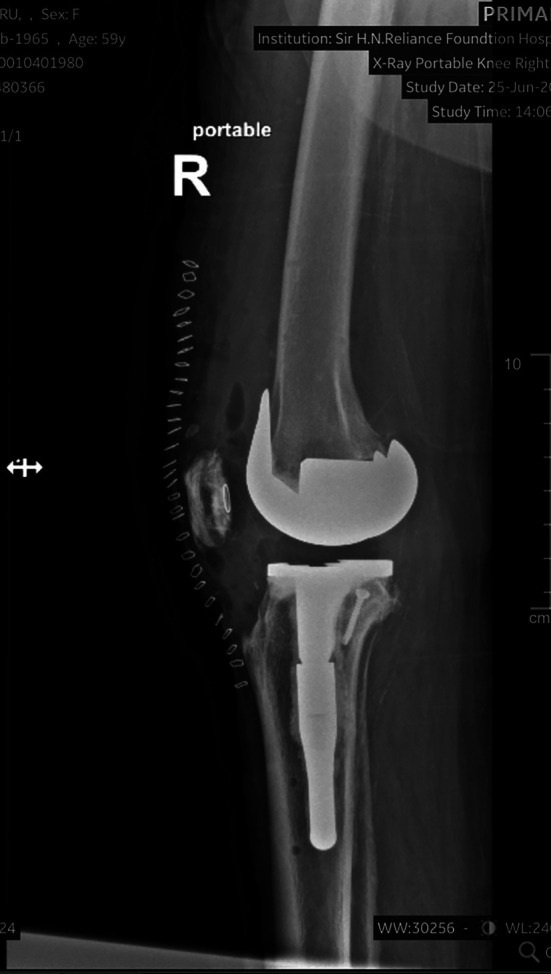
Postoperative radiographs showing defect managed with stem and screw.
